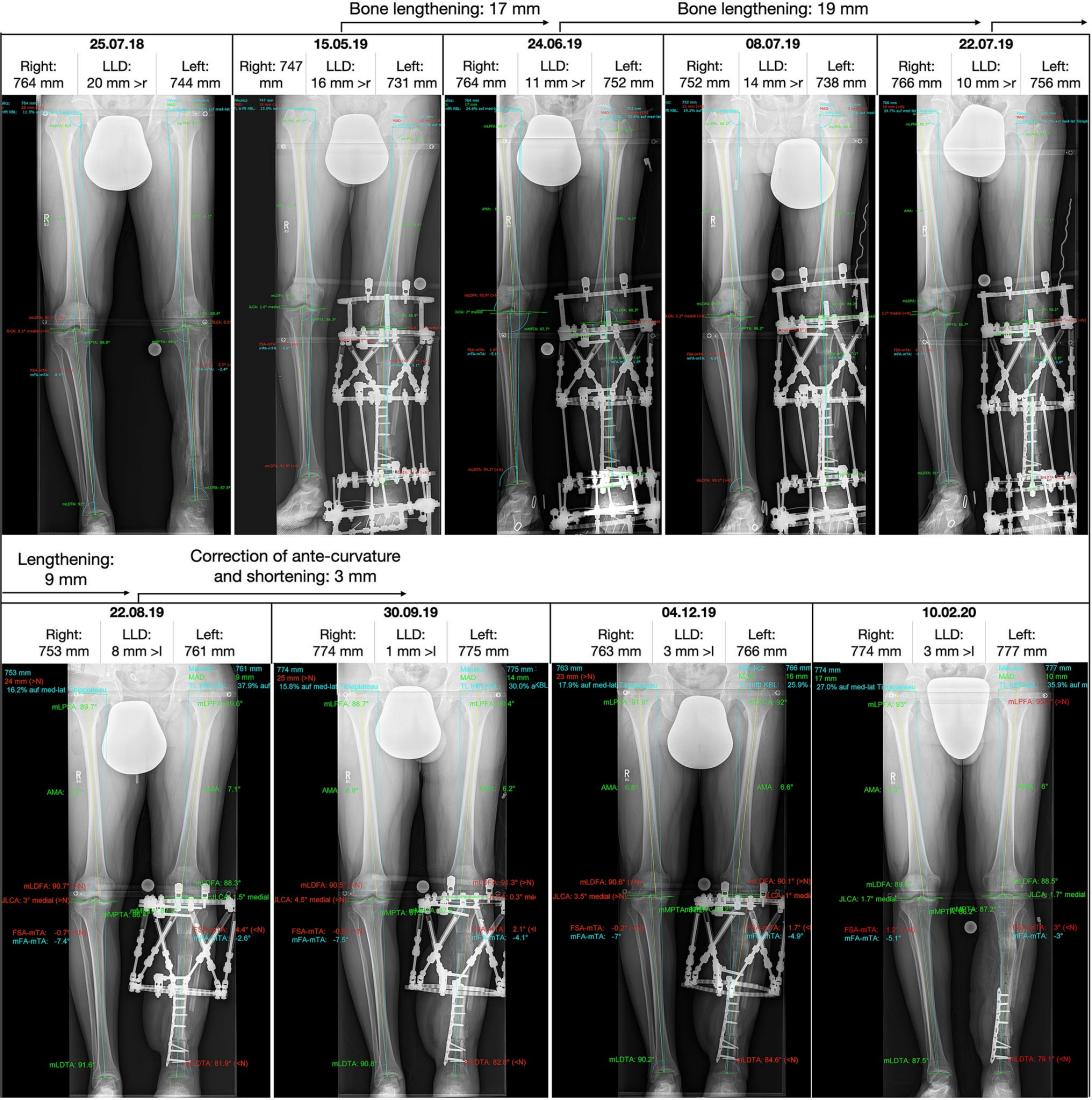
Example of the variability of limb length within one patient during bone lengthening. The length of the untreated right leg varies between 747 and 774 mm. Bone lengthening protocols had to be re-calculated due to variability of limb length of both legs on long-leg radiographs leading to prolonged treatment time (approx. 3 months) (*mFA-mTA* mechanical tibiofemoral angle, *MPTA* medial proximal tibia angle, *mLDFA* mechanical lateral distal femur angle, *mLDTA* mechanical lateral distal tibia angle, *mLPFA* mechanical lateral proximal femur angle, *JLCA* joint line convergence angle, *AMA* anatomical and mechanical femoral angle)
